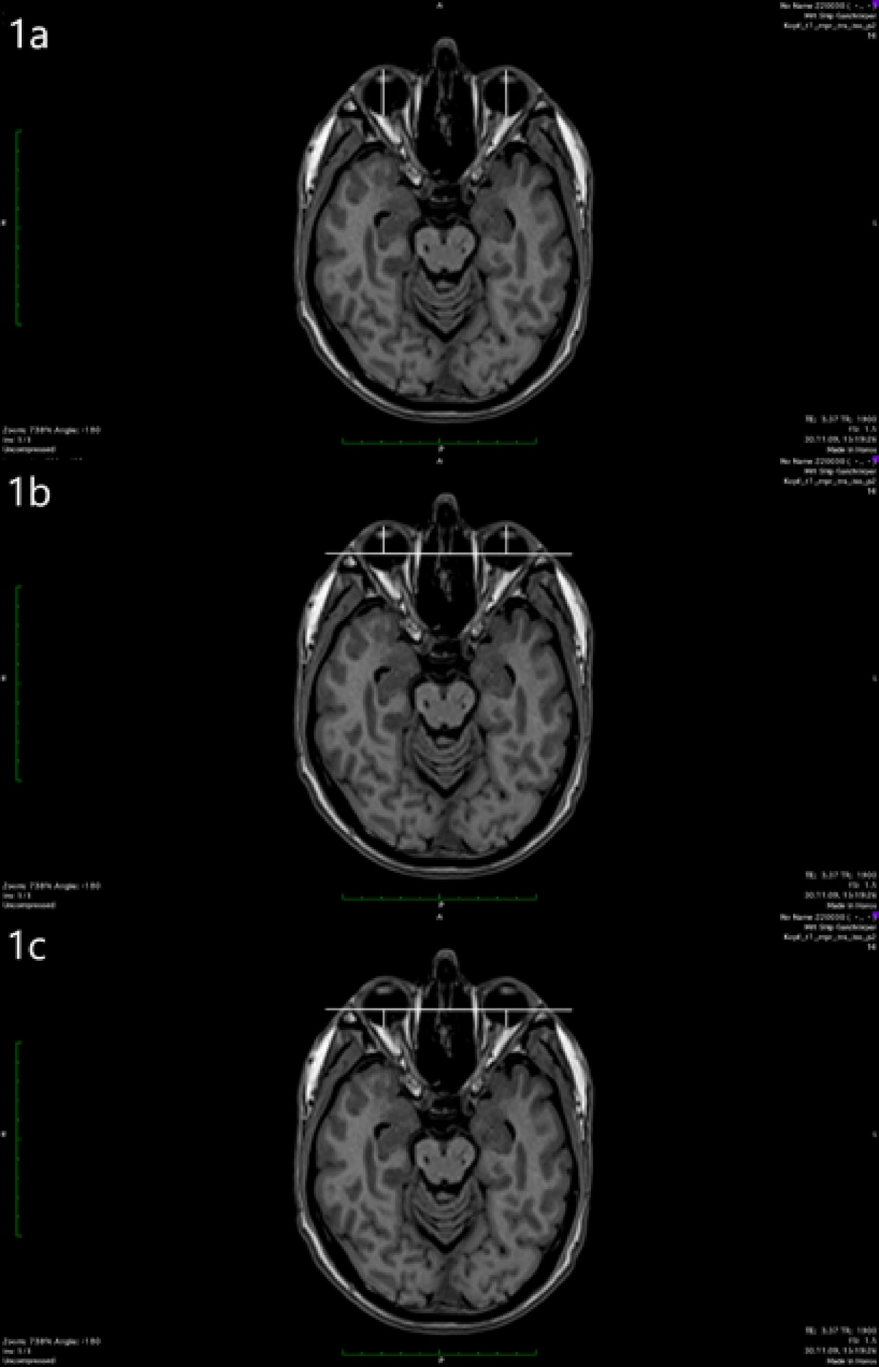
(1a) Bulbar axial length, (1b) Anterior exophthalmometry, (1c) Posterior exophthalmometry of the eye in an axial T1-weighted MRI.
